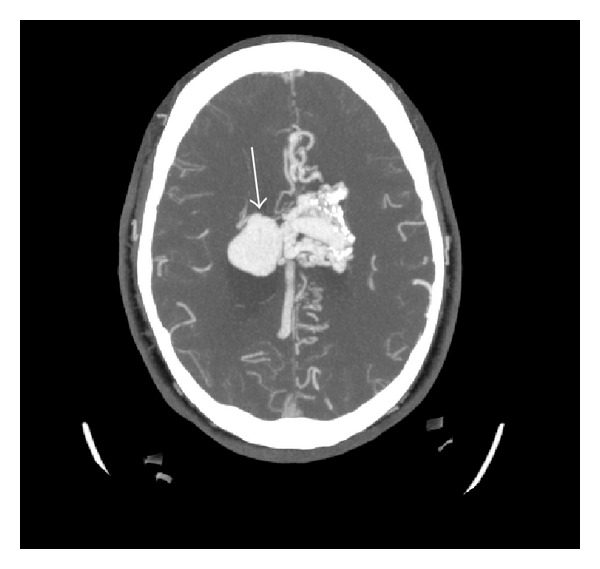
Axial contrast enhanced CTA (MIP) of the brain demonstrating a serpiginous intraventricular enhancing structure (arrow).
